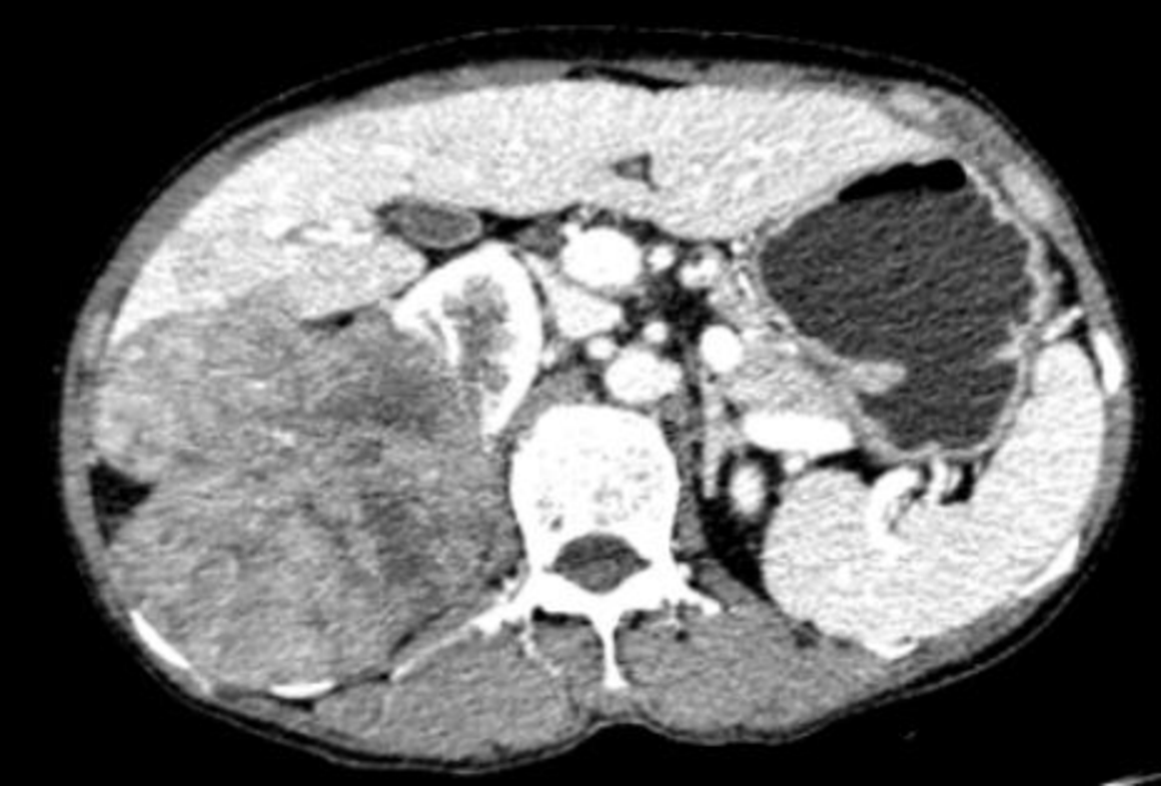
Abdominal CT, March 7, 2025: Multiple hepatic lesions, suggestive of hepatocellular carcinoma. The largest lesion measured approximately 9.3 cm × 9.1 cm at its larger cross-section, demonstrating protrusion beyond the liver to invade the right kidney.
